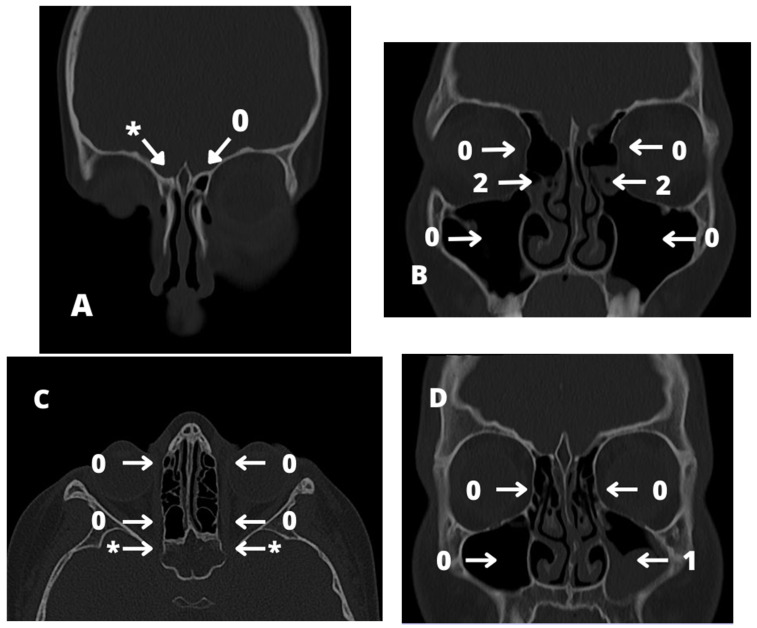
Examples of computed tomography (CT) findings of the paranasal sinuses and assessment of inflammatory disease severity using the Lund–Mackay scoring system. (A)—aplasia of the right frontal sinus (marked with *), (B)—bilateral obstruction of the osteomeatal complexes, (C)—aplasia of both sphenoid sinuses (marked with *), (D)—moderate inflammatory changes within the left maxillary sinus.
